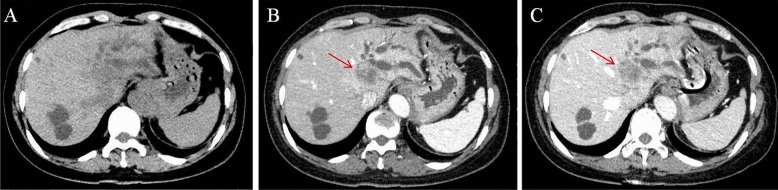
(Patient 1) Abdominal contrast-enhanced CT scan reveals a 2.8 * 2.5 cm slightly low-density circular lesion in the left lobe of the liver. Mild enhancement is seen at the lesion edges during the arterial phase, while mild infiltration of the contrast agent leads to slightly lower-density appearance in the portal venous phase. Adjacent left hepatic lobe shows bile duct dilation. Images (A), (B), and (C) correspond to the non-contrast, arterial, and venous phases, respectively.
